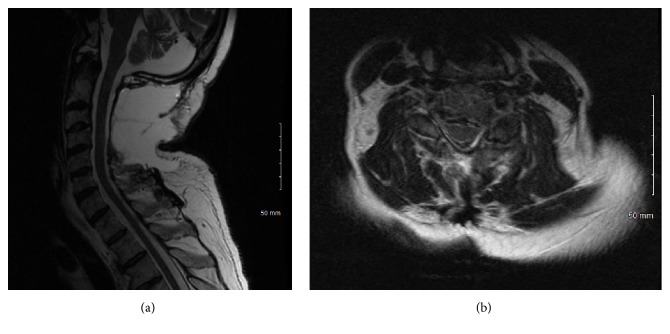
Postoperative T2-sequence MRI of the cervical spine. Sagittal (a) and axial (b) images, showing complete resolution of the preoperative intramedullary lesion and its surrounding T2-signal changes.
